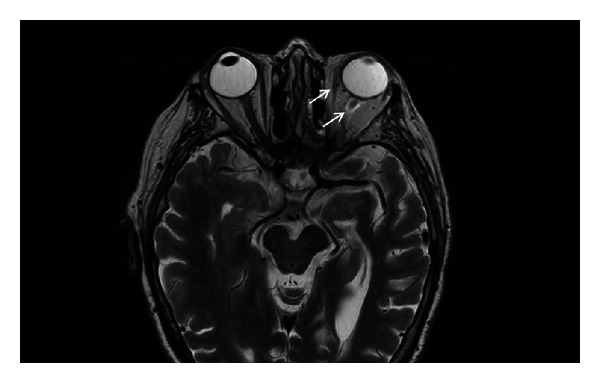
Orbital MRI. Orbital MRI revealed mildly elevated signal within the intra-orbital segment of the left optic nerve, mild inflammatory change surrounding the optic nerve sheath and very mild asymmetric prominence of the left extra-ocular muscles concerning for myositis (arrows).
